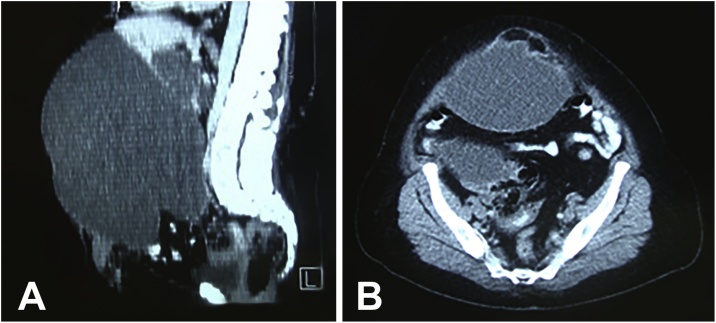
A: CT showed a giant bile duct cyst. B: CT confirmed that epigastric hernia content contained part of the cyst.
